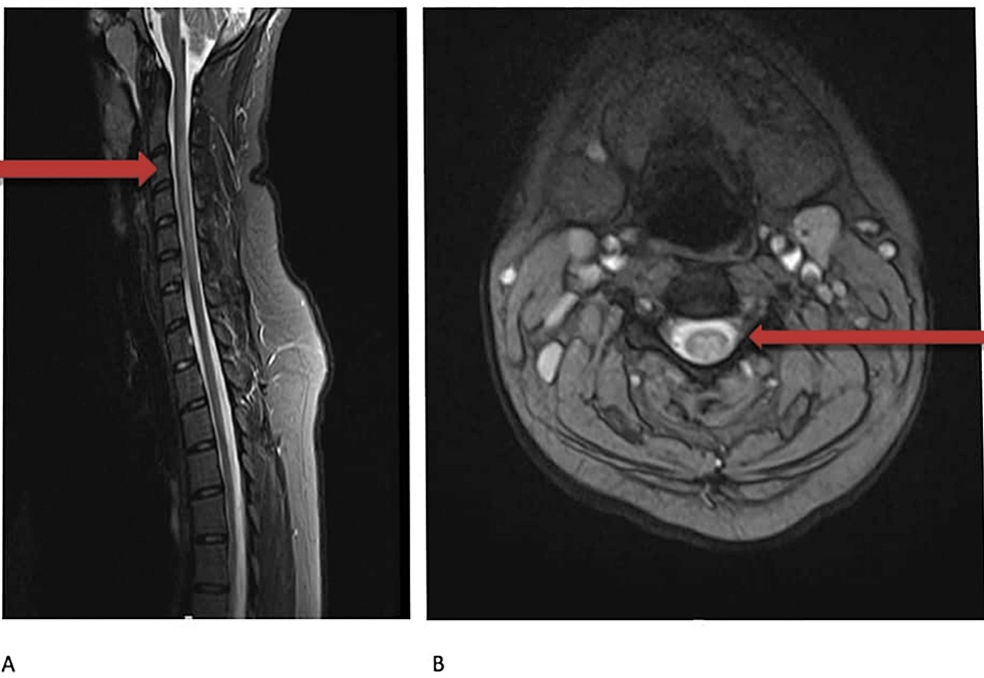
(A, B) MRI of the cervical spine demonstrating bilateral symmetrical T2 high signal changes in the dorsal columns extending from C2 to C6 level.
